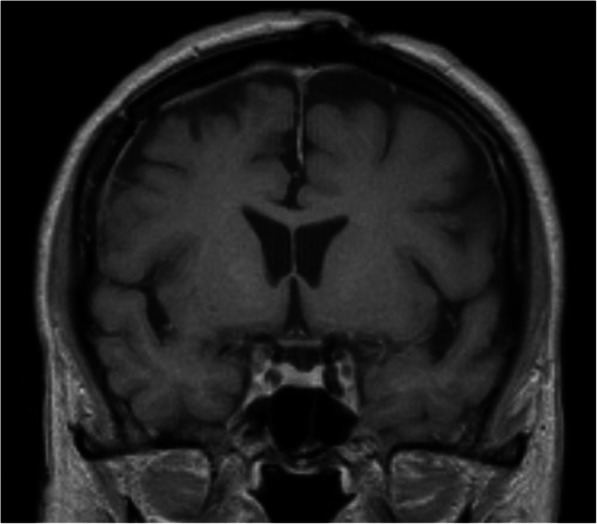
Postoperative MRI. T1 weighted image, coronal section. No residual or recurrent tumor mass
